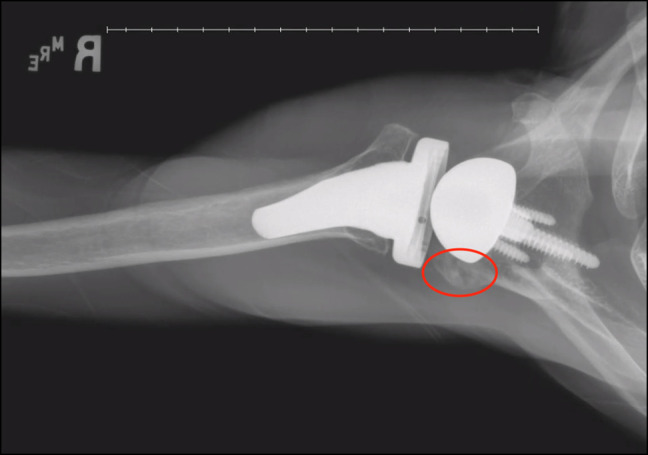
Radiograph of patient 1 after intranasal calcitonin treatment for a Levy II acromial fracture.
